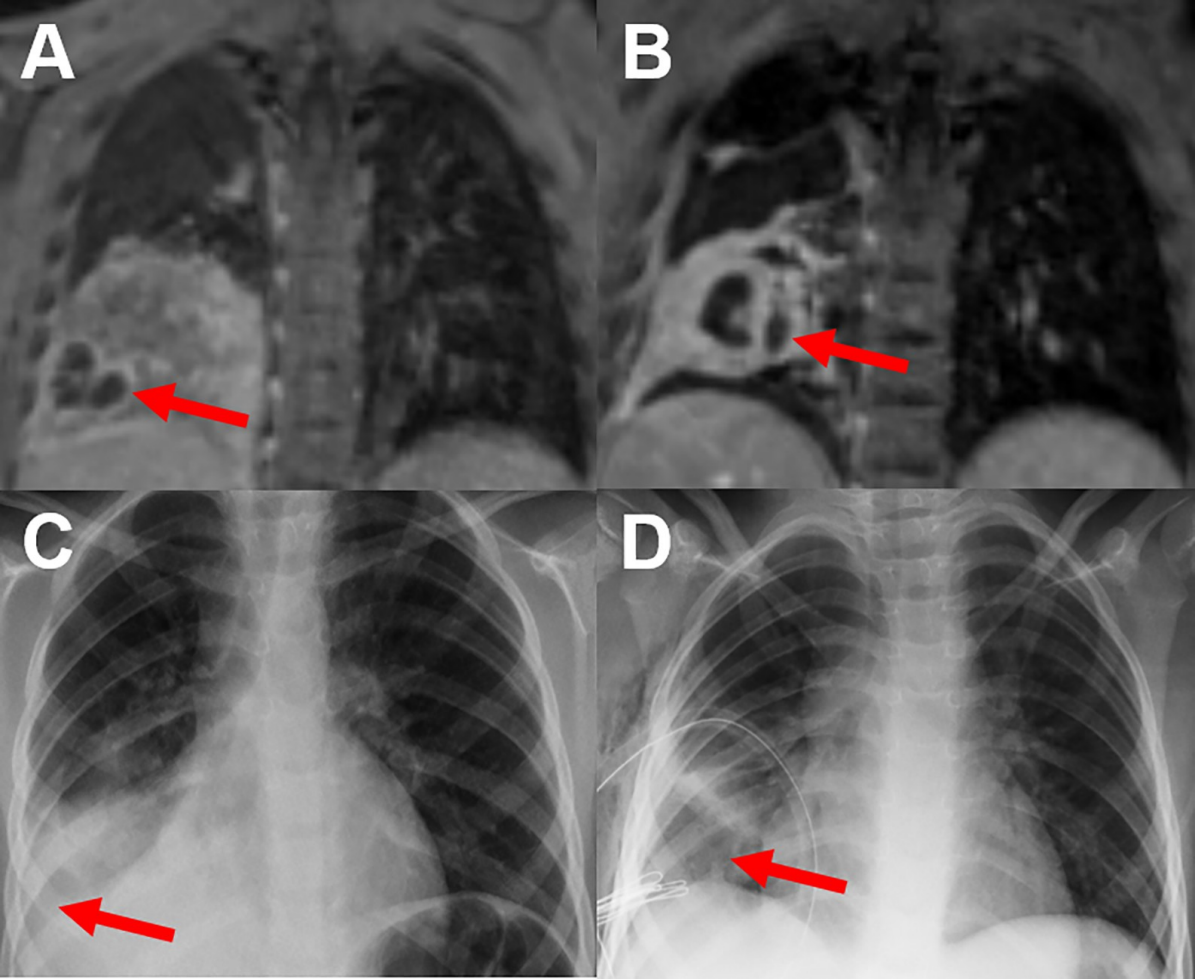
Comparison of T2-weighted sequences and CXR of an 11-year-old child at baseline and follow-up. MRI shows an abscess formation in the right lower lobe at baseline (red arrow) (A). The surrounding inflammatory changes regressed under therapy, whereas the abscess formation increased at follow-up (red arrow) (B). The abscess formation was not detected by CXR (red arrows) (C+D). Furthermore, the extension of pathologic findings was underestimated by CXR (D).
